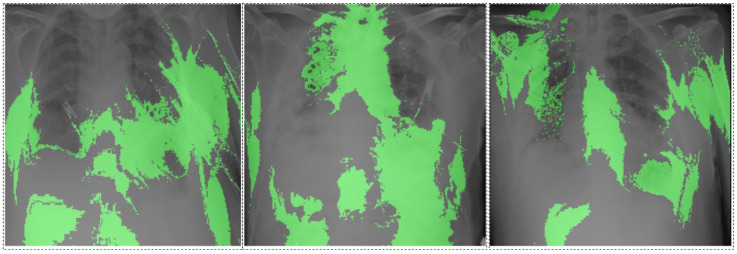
LIME explanation of key X-ray regions.
